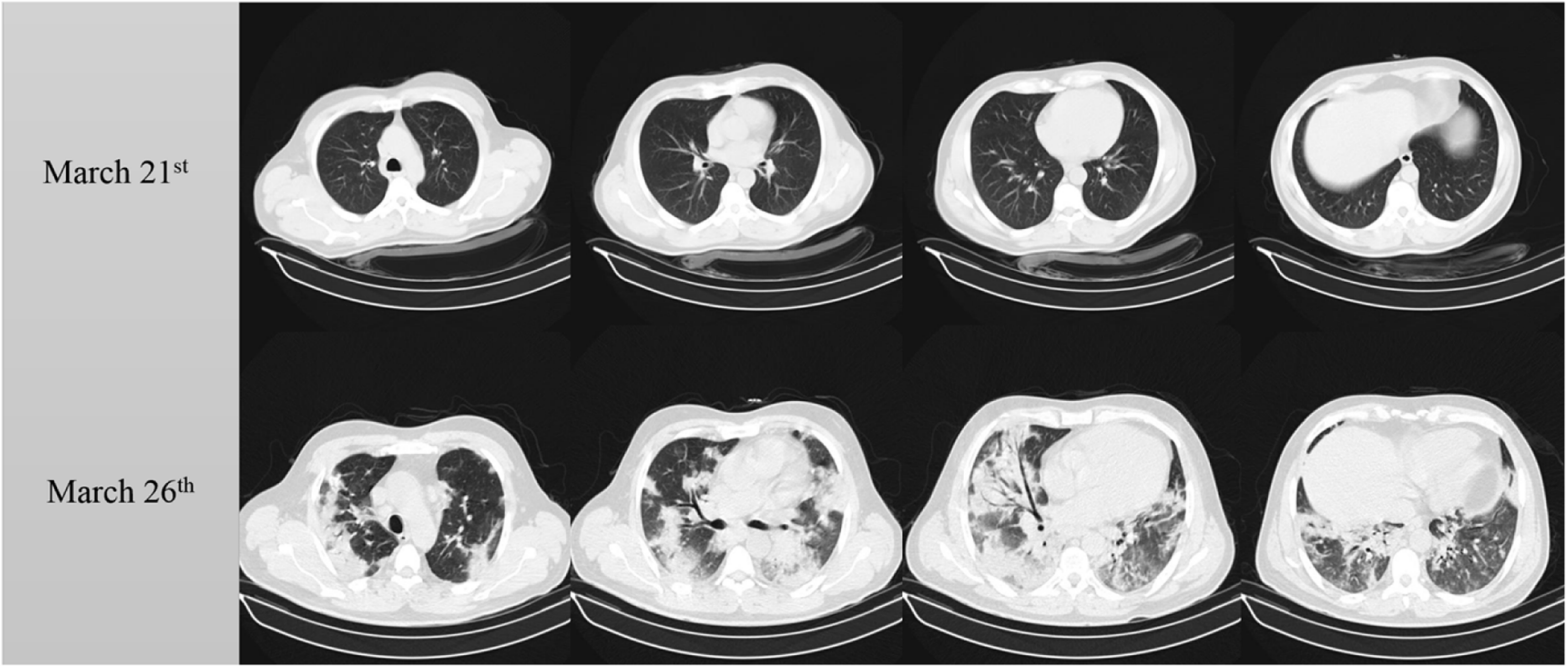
The first row is the chest CT which is obtained at the first presentation of the patient to the emergency ward, and the second row is the chest CT which is obtained days after. As it is obvious, the first CT scan shows the normal appearance, and the following one shows multiple bilateral ground-glass opacities with airspace consolidation and some degree of bronchiectasis in the lower lobes
